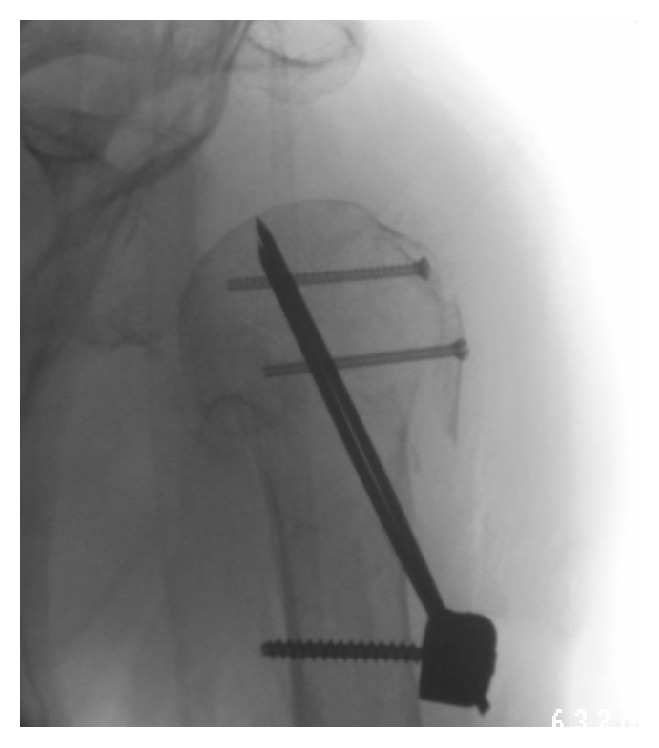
Intraoperative X-ray after reduction and stabilization using the Humerusblock.
